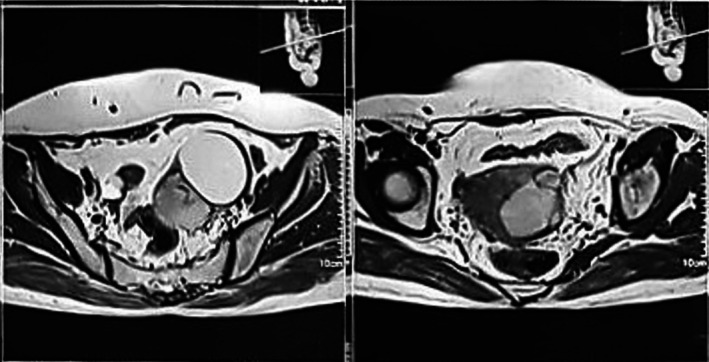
Patient's presurgical MRI of the abdomen and pelvis revealed a 7.4 × 7.3 × 5.7 cm cystic and solid lesion in the left adnexa extending into the left ovary and uterine wall, and a 3.6 × 3.3 × 1.7 cm cystic and hemorrhagic lesion in the right adnexa extending into the right ovary and the right uterine wall.
